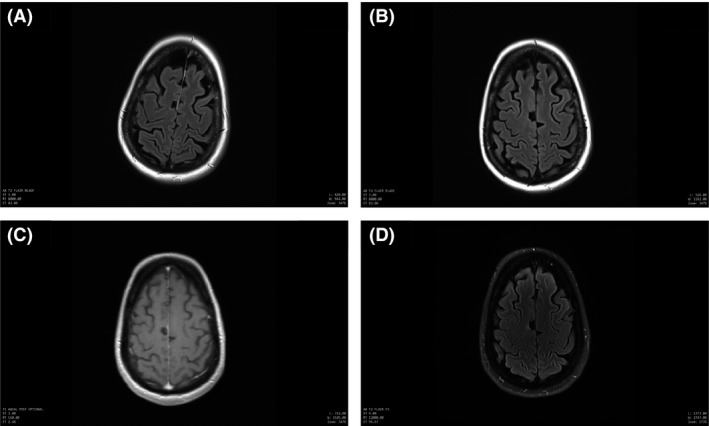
MRI of the brain after the lesionectomy. (A) Seven months after onset of EPC, T2 FLAIR sequence shows an area of encephalomalacia over the right frontal cortex in the region of surgery. (B) Twelve months after onset of EPC, T2 FLAIR sequence shows stable postsurgical changes over the right frontal cortex in the region of surgery despite the worsening of EPC. (C) Twelve months after onset of EPC, T1 postcontrast sequence shows no contrast enhancement around the area of encephalomalacia. (D) Two years after onset of EPC, T2 FLAIR sequence shows stable postsurgical changes. In addition, no asymmetric brain volume loss was noted.
